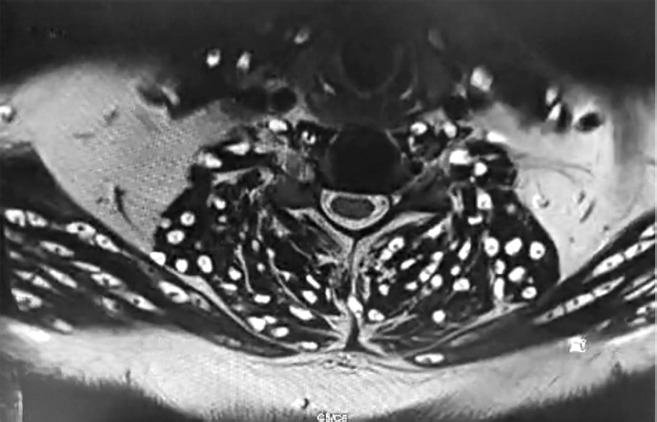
Description: Axial T2-weighted MRI at the C5–C6 level demonstrates numerous hyperintense cystic lesions embedded within the paravertebral and posterior cervical musculature. Several lesions reveal an eccentric mural nodule corresponding to the scolex, characteristic of myocysticercosis. The spinal cord appears preserved in contour and signal intensity, with no evidence of intramedullary pathology or compressive myelopathy. Clinical Significance: This axial section confirms advanced muscular involvement by cysticercosis at the mid-cervical level. The demonstration of scolex within multiple cysts provides a definitive diagnosis, separating it from other cystic or inflammatory muscular lesions. Preservation of spinal cord integrity and absence of myelopathy are reassuring findings that emphasize systemic medical management as the primary treatment approach. Origin: @GSVM Medical College, PMSSY Superspeciality Hospital, Kanpur, Uttar Pradesh, India. Pincode: 208002.
